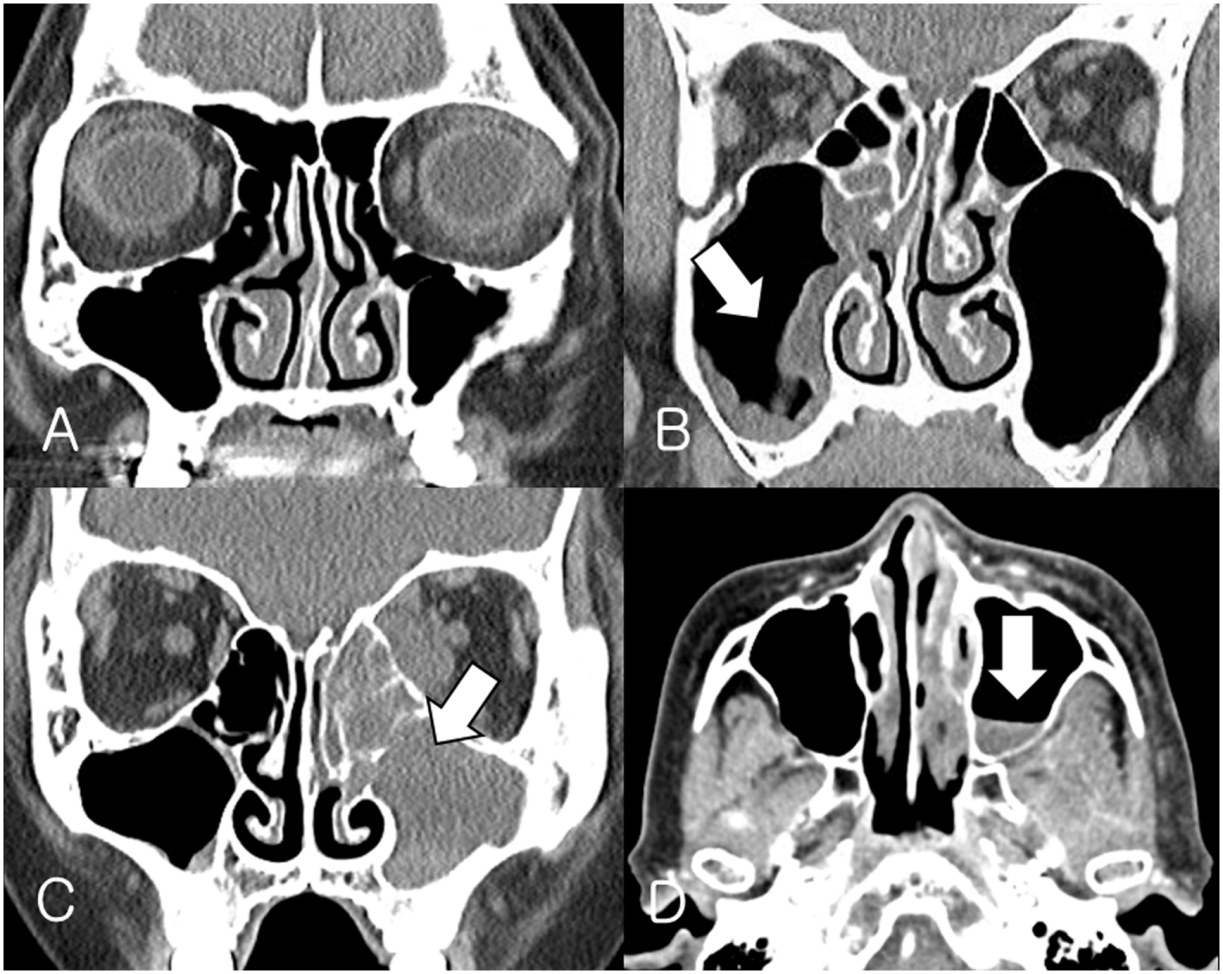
Computed tomography findings of rhino-orbito-cerebral mucormycosis and bacterial orbital cellulitis. A. Clear maxillary and ethmoid sinuses; B. mucosal thickening in the right maxillary sinus; C. full opacification in the left maxillary and ethmoid sinuses; and D. air-fluid level in the left maxillary sinus.
